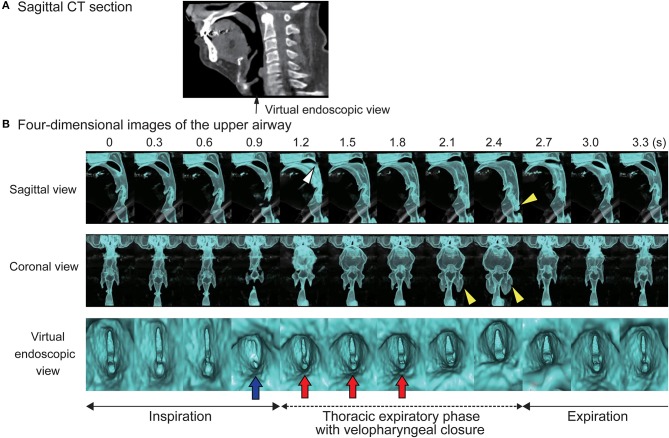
Four-dimensional computed tomographic (4D-CT) images. (A) Sagittal section of computed tomography. (B) Serial four-dimensional computed tomographic images of upper airway during sleep shows glottis closure at a terminal stage of inspiration (blue arrow), subsequent velopharyngeal closure (white arrow head), expansion of the pharyngeal cavity (yellow arrow heads), and succeeding glottis opening with increase of pharyngeal pressure (red arrows).
